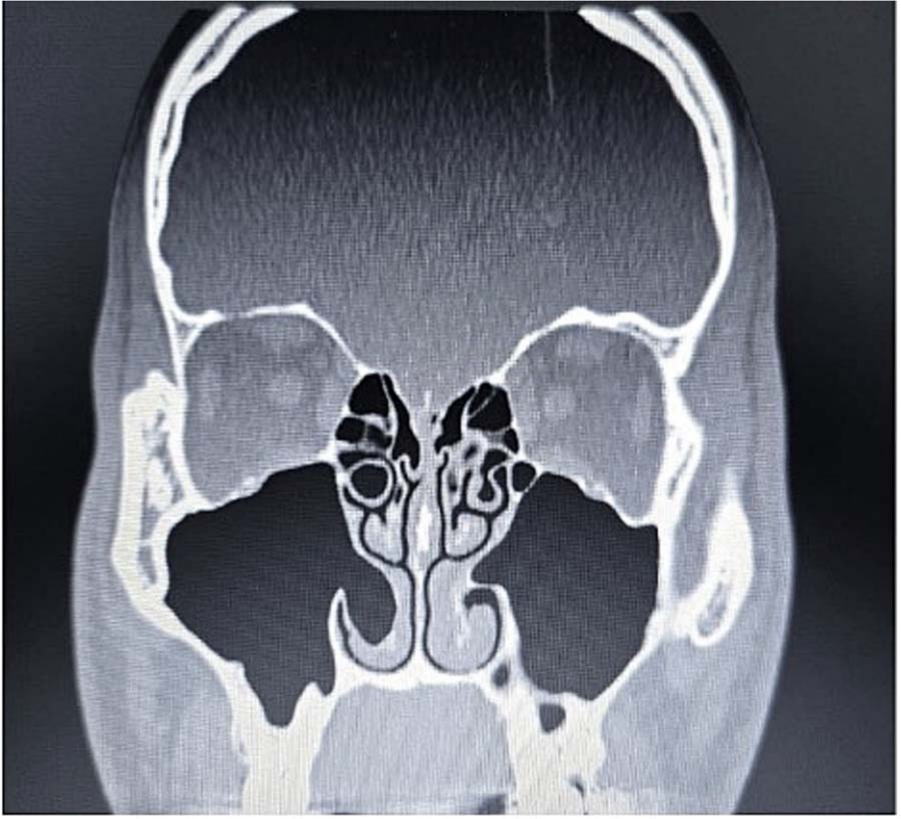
Paranasal sinus CT scan in the coronal plane demonstrating bilateral inferior concha bullosa with communication to the maxillary sinuses.
